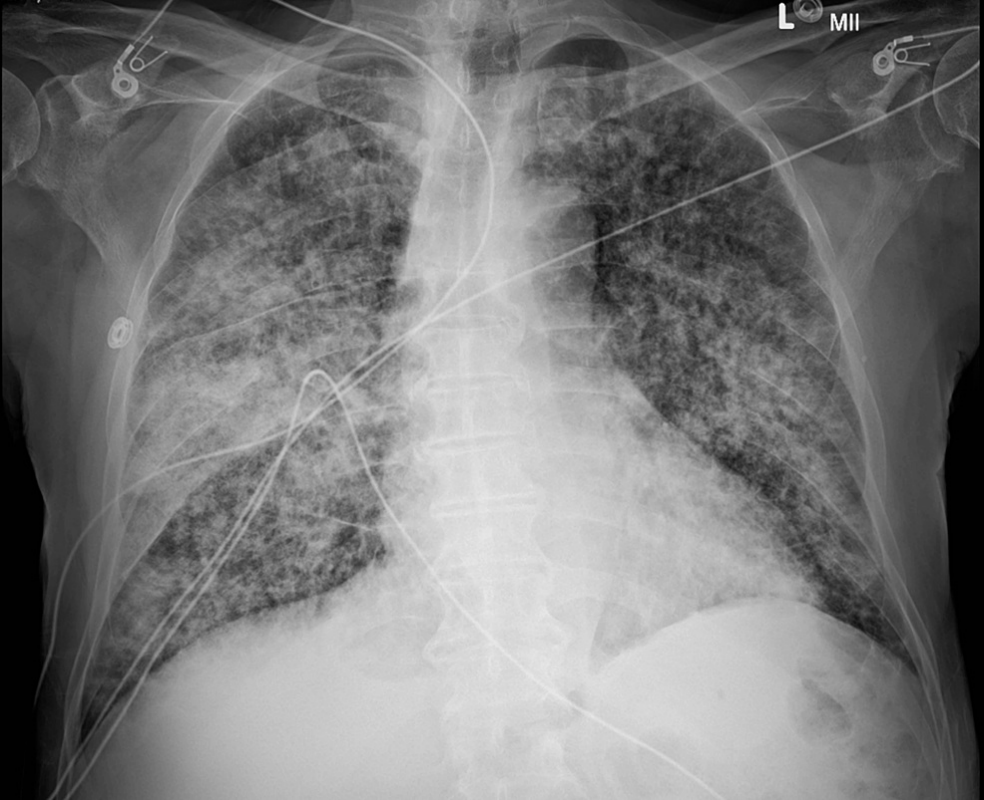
Portable semi-upright anteroposterior chest X-ray demonstrating a diffuse reticulonodular pattern with denser consolidation in the right mid-lung field.
